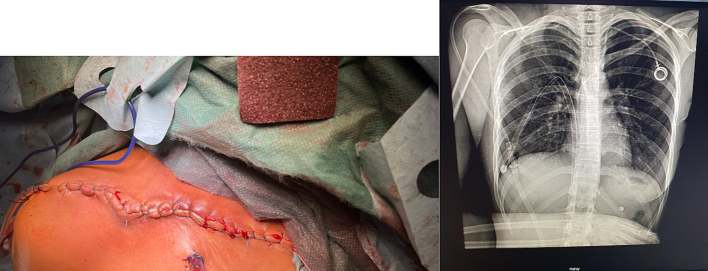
Control X-ray taken on day 1 postop with the chest drain still in place.
